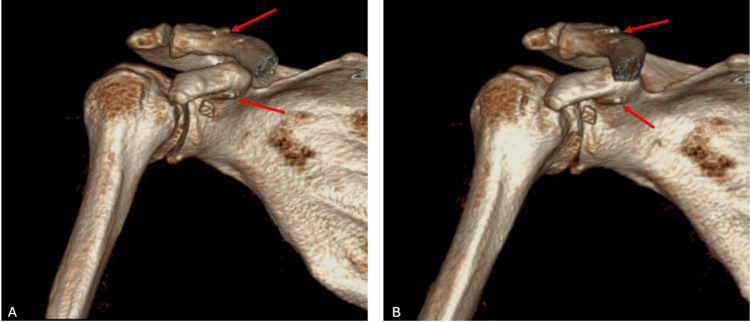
Postoperative 3D reconstructed thin-slice CT images of the patient The images show the tunnel placement in the coracoid bone and clavicle, confirming proper implant positioning within the tunnel. The red arrows indicate the EndoButtons.
